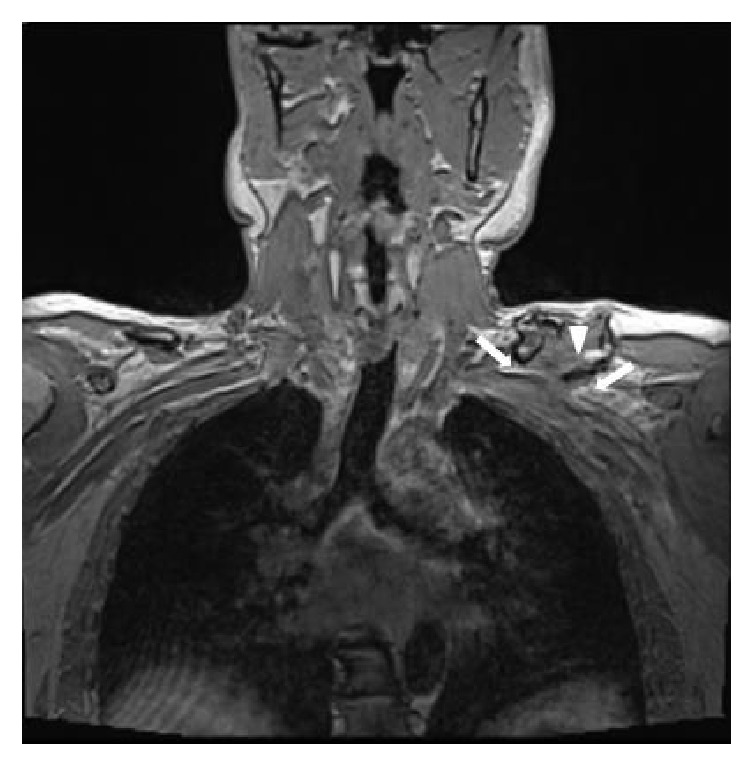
Coronal three-dimensional T1-weighted volumetric interpolated breath-hold image shows compression of the infraclavicular part of the brachial plexus (white arrow) by an inferior bone fragment (white arrowhead).
